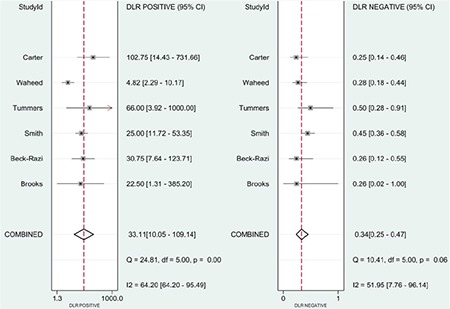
Summary of positive likelihood ratio and negative likelihood ratio of focused assessment with sonography in trauma for assessing injury period.
